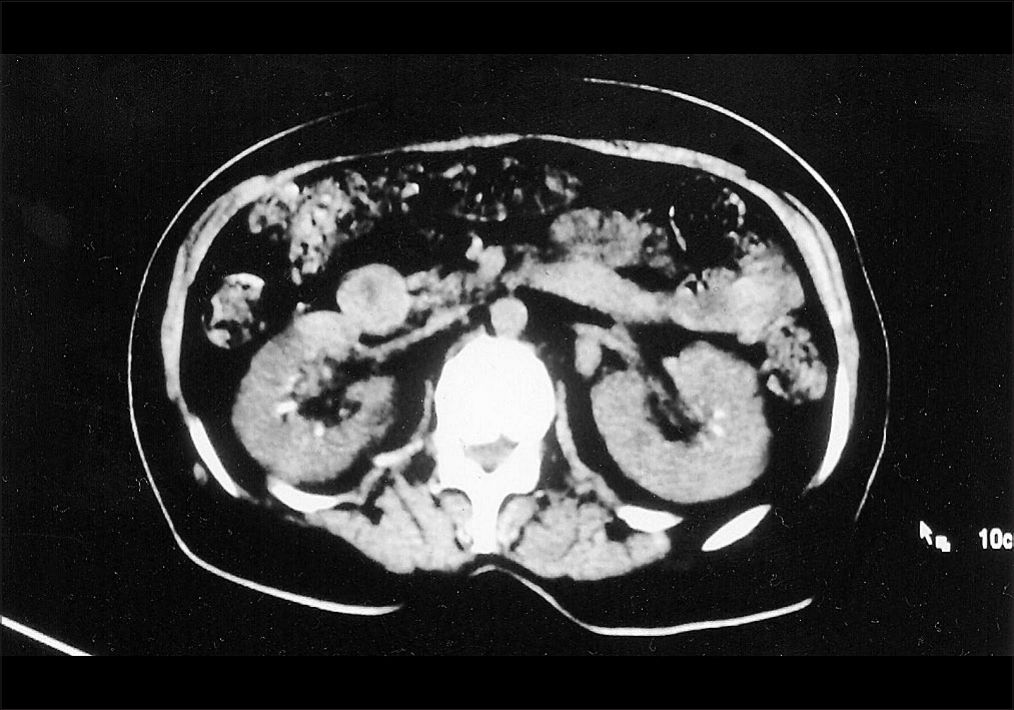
Unenhanced computed tomography showing multiple calcific densities in the segmental renal artery and in its branches on both sides
